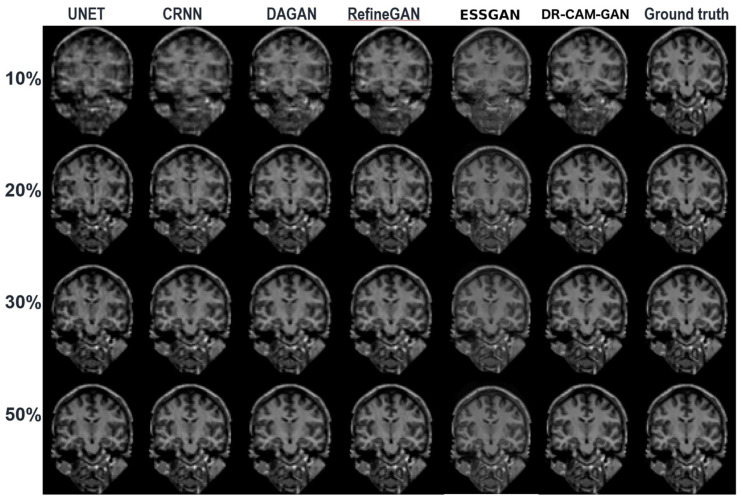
A coronal slice reconstructed from a T1-weighted MPRAGE volume by five distinct DL models (column-wise) at four different CS undersampling rates (row-wise).
